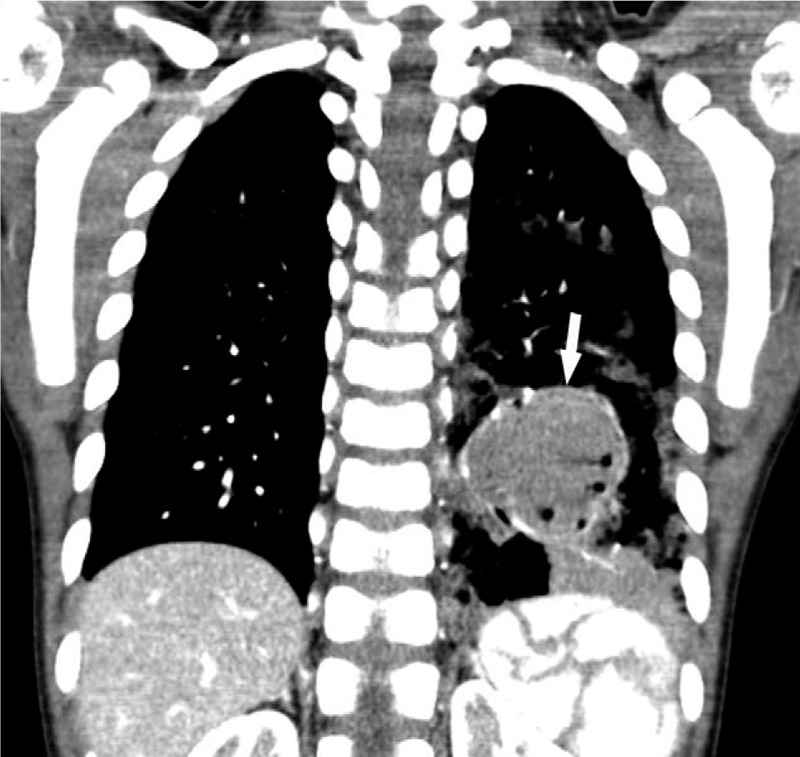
Contrast-enhanced chest computed tomography (CT). A 4.5 cm × 3.4 cm sized cystic pulmonary airway malformation is seen in left lower lobe (arrow).
